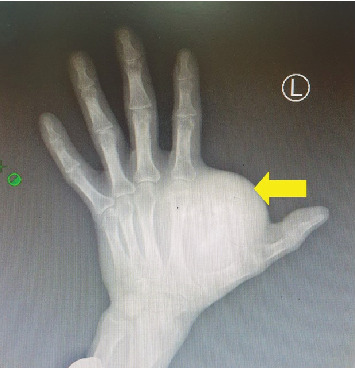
X-ray left hand showing a soft tissue mass (yellow) between first and second metacarpals with areas of calcification with no bone erosion.
